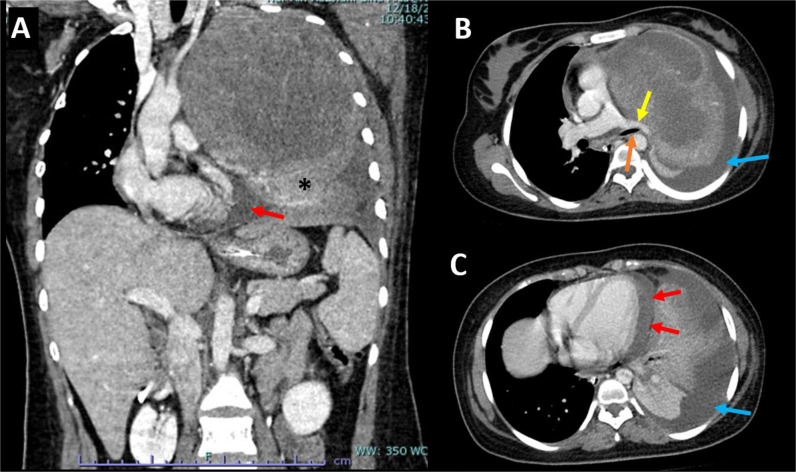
Contrast-enhanced computed tomography of the thorax in coronal (A) and axial (B and C) planes shows a large enhancing mass with central necrosis occupying the left hemithorax. It exerts mass effect, causing contralateral mediastinal shift, collapse, inferior displacement of left lower lobe (*), and narrowing of the left main bronchus (orange arrow) and the left pulmonary artery (yellow arrow). Pericardial (red arrows) and left pleural effusions (blue arrows) are present. The right hemithorax and upper abdominal organs are unremarkable.
